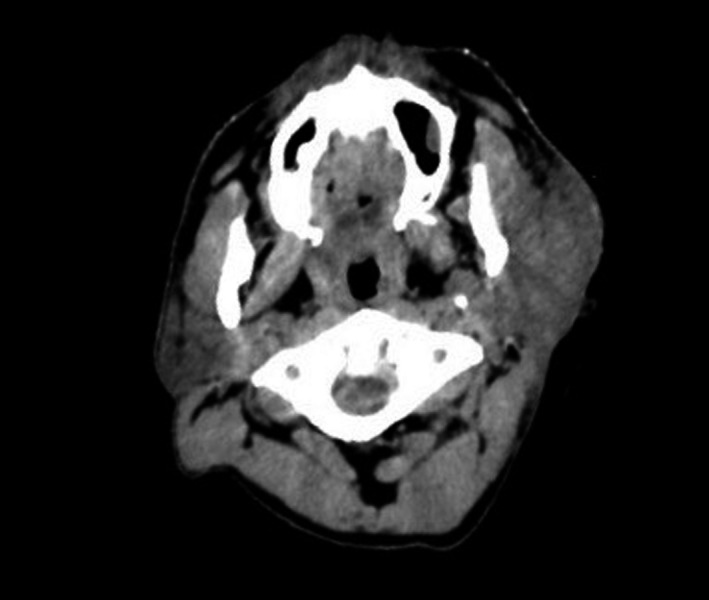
Axial planning CT showing diffuse infiltration of the left parotid and subcutaneous soft tissues (anatomical distortion due to rotated head position for electron treatment).
